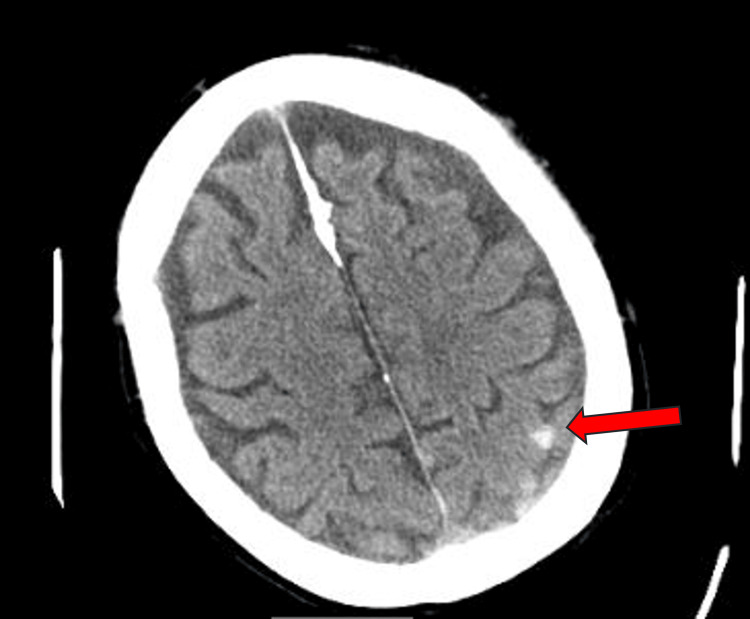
Patient 2 head CT without contrast occipital lobe hemorrhage
